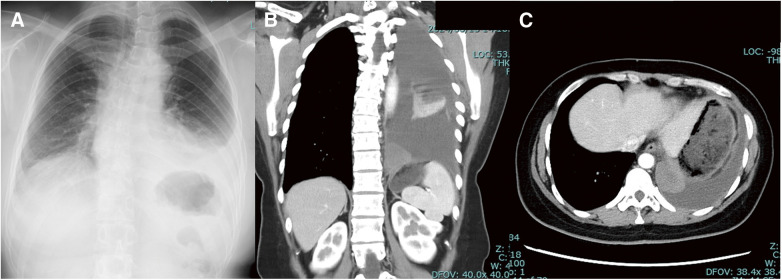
Radiographic findings before surgery. Left pleural effusion and left atelectasis are noted (A). A mass lesion with a maximum diameter of 4.5 cm is observed on the left side at the Th11 level (B). The contrast-enhanced effect of the mass is rarely observed from the arterial phase to the late phase. It is presumed that the area around the mass is located within the mediastinum, with a thickened pleura (C).
